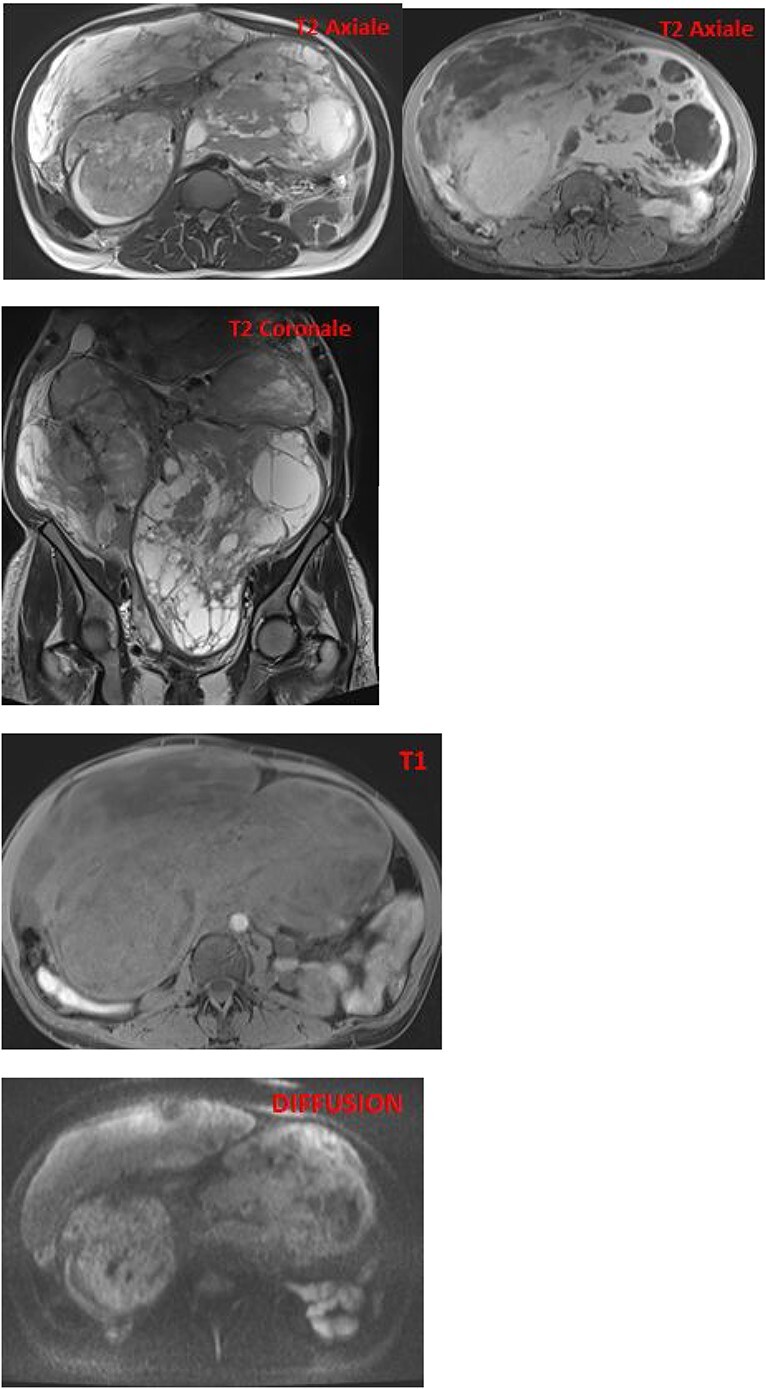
MRI images reveal an enlarged uterus with multiple myometrial formations exhibiting intermediate signal on T1 and T2, encompassing regions of hyperintensity on T2 and hypointensity on T1, enhanced homogeneously compared with the myometrium and without diffusion restriction, the most characteristic ones are classified as FIGO 6, FIGO 7, and FIGO 2–5, suggesting uterine leiomyomatosis with cystic degeneration.
